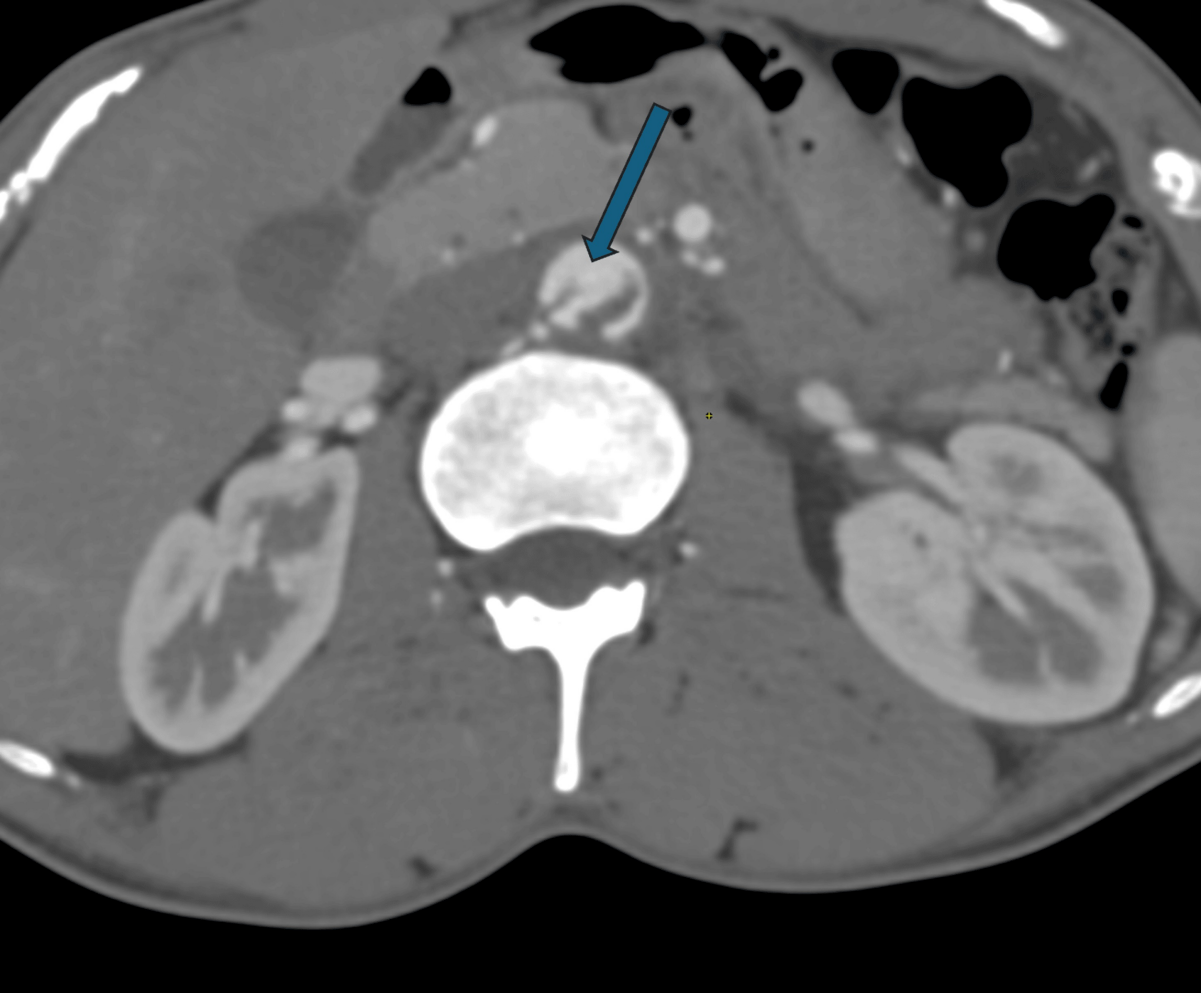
CECT abdomen axial section showing discontinuity in the dissection flap (shown by thick arrow) CECT: contrast-enhanced computed tomography
